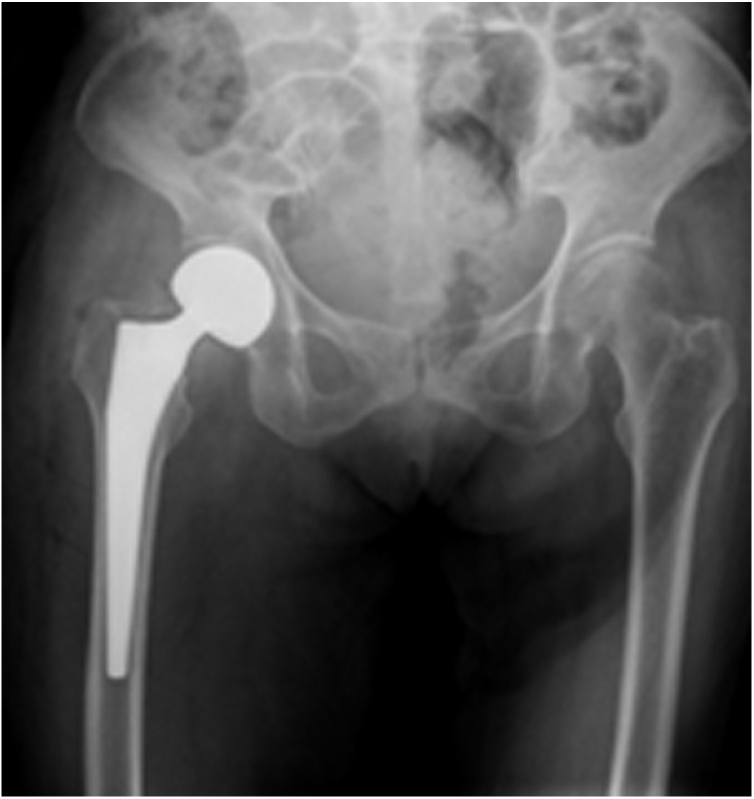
Anteroposterior radiograph of the right hip demonstrating a satisfactory hip hemiarthroplasty subsequent to a subcapital neck of femur fracture.
